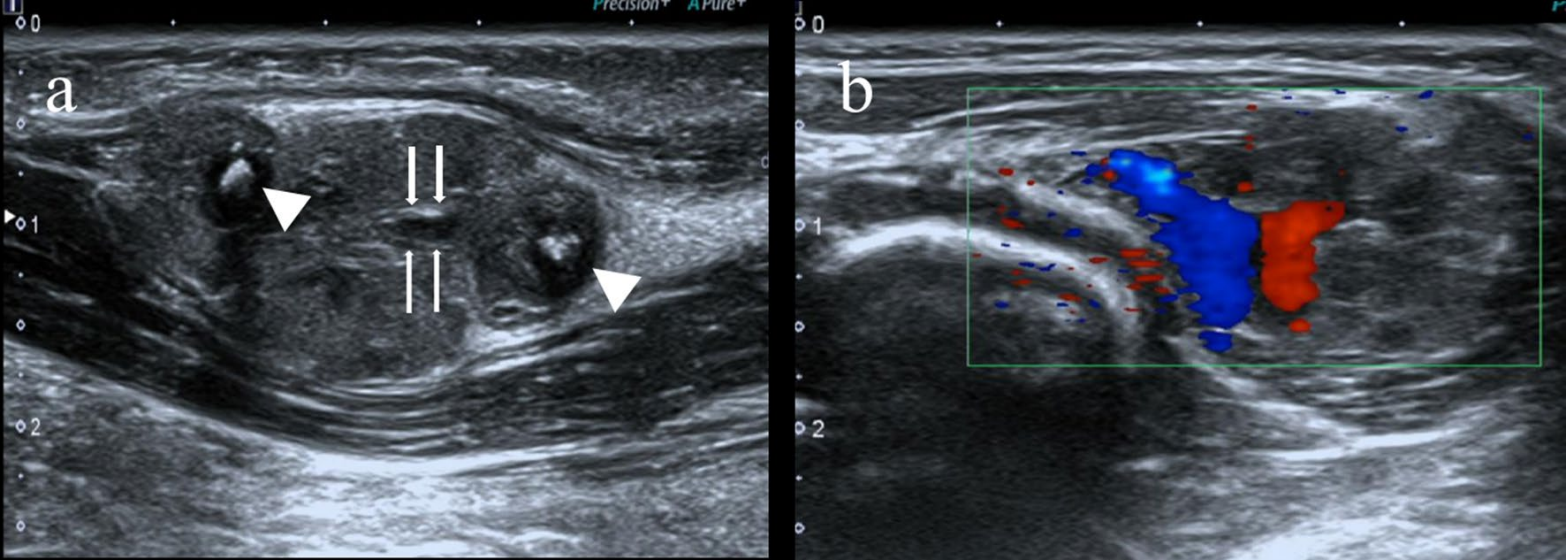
Asymptomatic intramuscular vein malformation was identified in a 3-year-old boy. (a) Transverse gray-scale US shows a slightly hyperechoic, reasonably well-defined mass located in the left upper arm biceps brachii with internal calcifications suspicious for phleboliths (arrowheads) and linear anechoic spaces (arrows) consistent with slow-flow venous channels. (b) Color Doppler flow imaging shows moderately central hyperemia.
